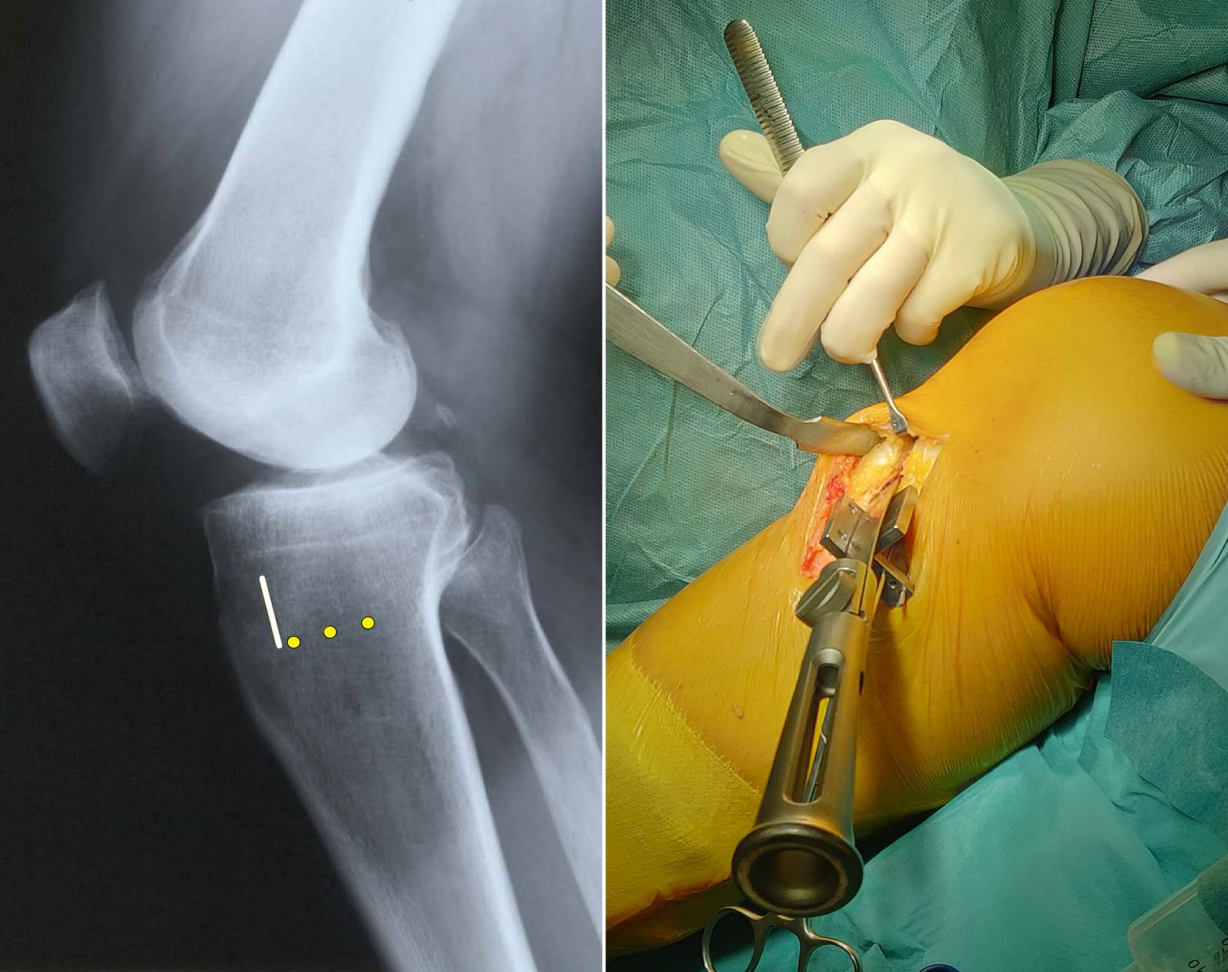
On the left, a graphical representation of the three guide wires (yellow dots) and the first coronal osteotomy (vertical white line), on a lateral X-ray view of a right knee. On the right, the intraoperative findings of the first coronal osteotomy completed with a sharp thin 2-cm large osteotome, in a right tibia, with the assistance of the self-made cutting guide
